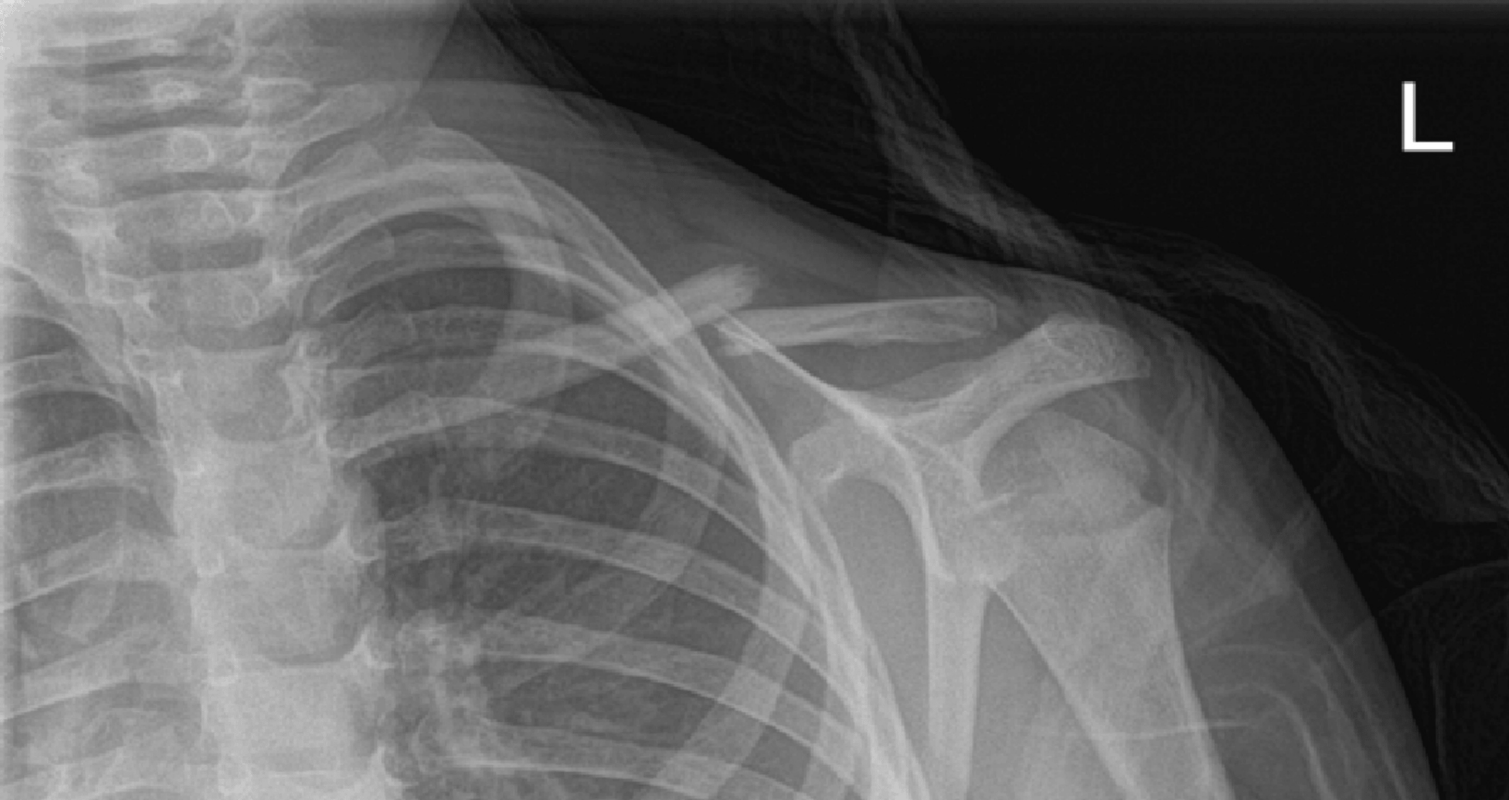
Anteroposterior radiograph demonstrating a displaced left middle third clavicle fracture (Case 2)
